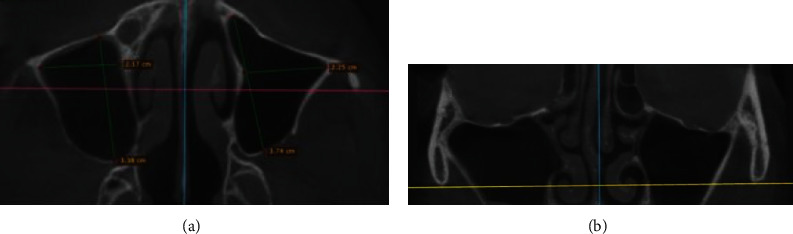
(a) Anteroposterior and mediolateral (1 and 3) measurements conducted along the root of zygoma level (AP ZG, ML ZG) on axial CBCT scan. (b) Coronal CBCT scan show the axial orientation axis (yellow horizontal line) denoting the level of axial scan along the root of zygoma.
